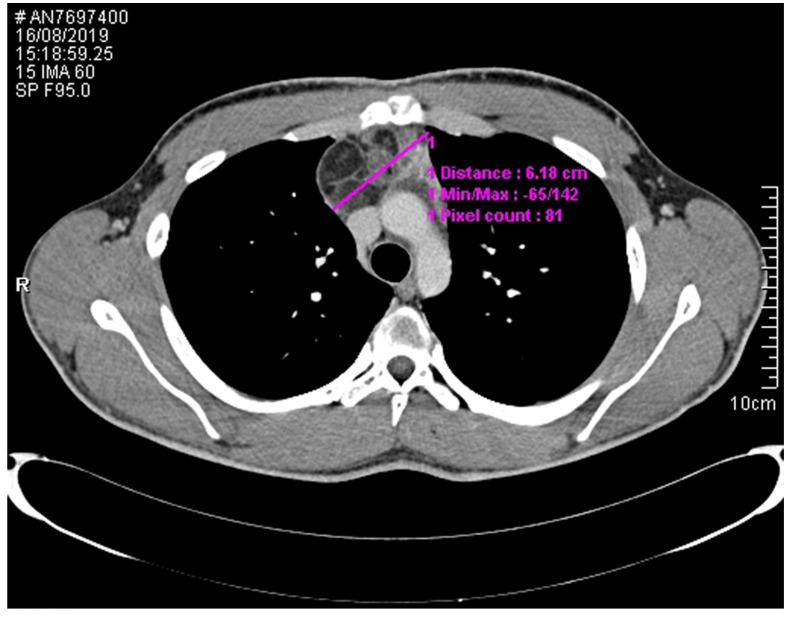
CT scan demonstrating a germinal cell tumor (GCT) in the anterior mediastinum.
